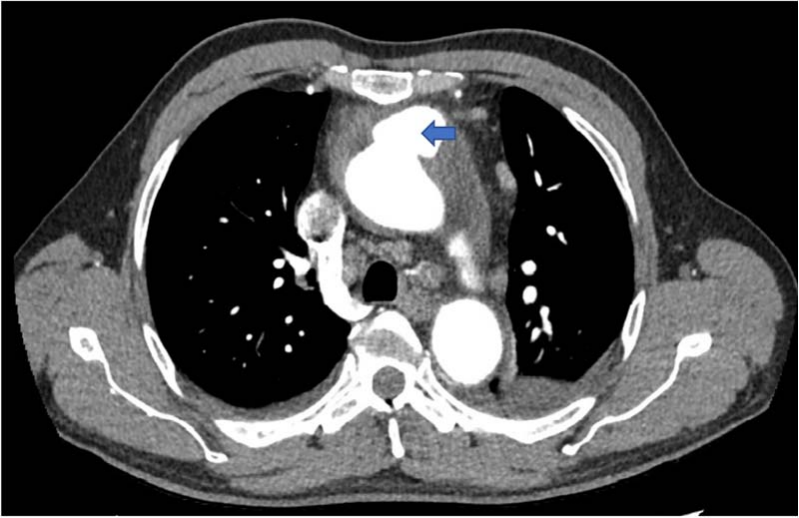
Axial CT image showing saccular aneurysm of the ascending aorta.
